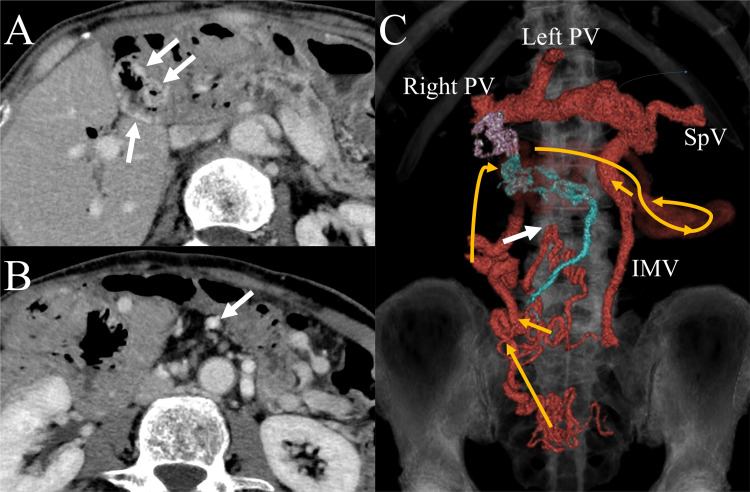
CT of the abdomen (A) Axial CT image of the upper abdomen showing jejunal varices (arrows) at the hepaticojejunostomy site. (B) Axial CT image 8 cm lower than (A) showing a continuous jejunal vein (arrow) with the jejunal varices. (C) Anterior view of three-dimensional CT showing the portal venous system. The varices (purple) are continuous with the jejunal vein (blue). The distal part of the SMV (white arrow) is visualized, but the proximal part of the SMV is occluded. Yellow arrows show the direction of the venous flow from the small intestine to the PV via the collateral vein and the inferior mesenteric vein. The central part of the collateral vein is made transparent to simplify the vascular anatomy presentation. SpV: splenic vein, CT: computed tomography, SMV: superior mesenteric vein, PV: portal vein, IMV: inferior mesenteric vein
